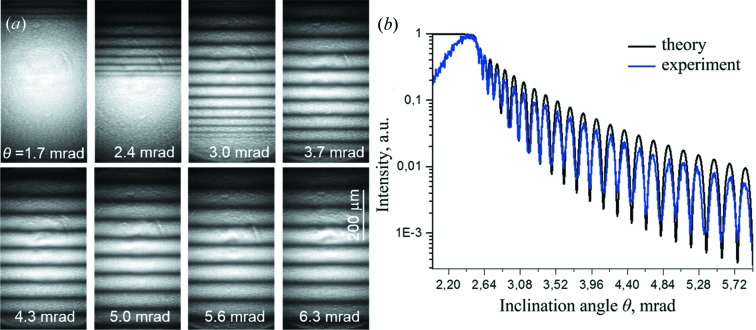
(*a*) Interference patterns registered for a 200 nm membrane at different inclination angles with 14.4 keV X-rays. The 200 µm scale corresponds to 448 µrad. (*b*) The experimental reflectivity curve obtained by combining interferograms recorded at membrane inclination angles from 2.4 mrad to 5.6 mrad.
